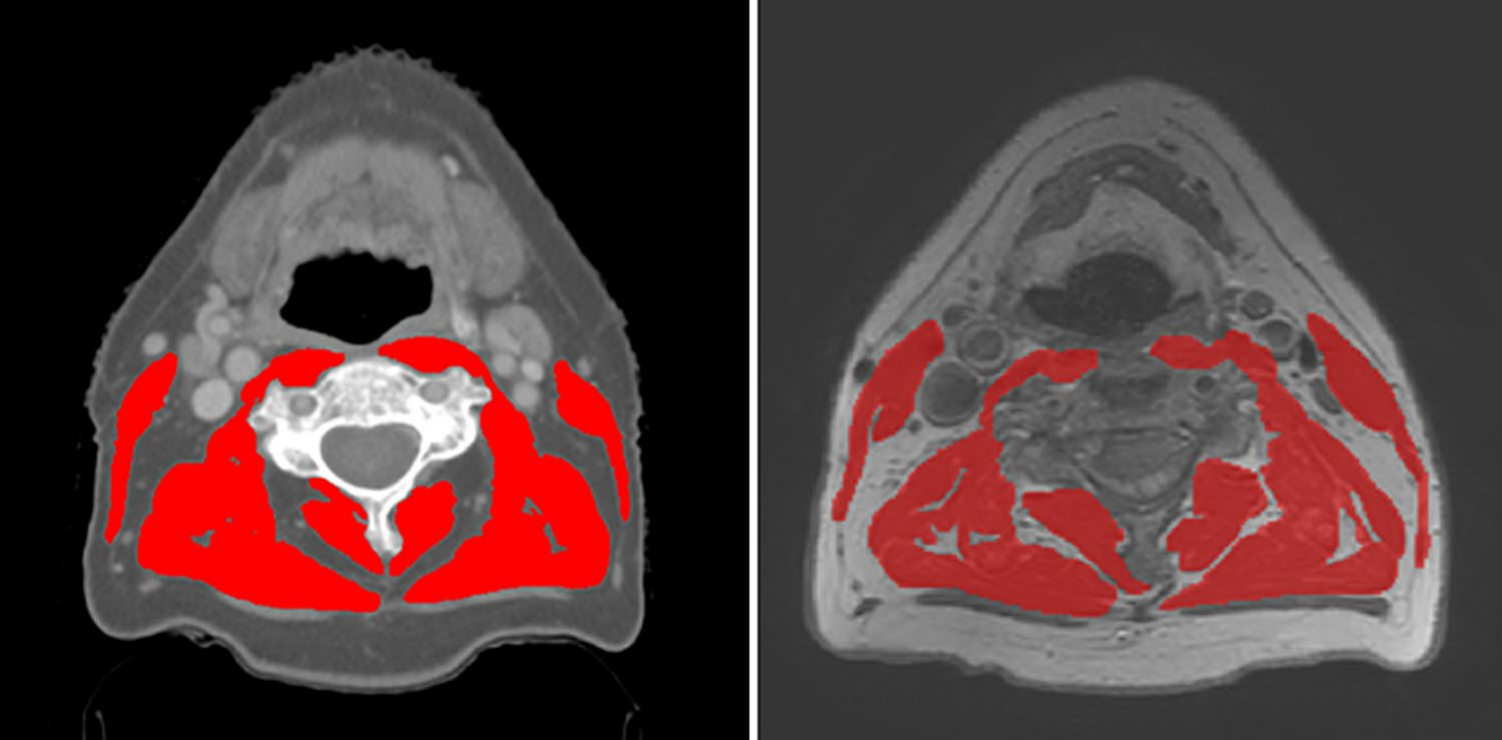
Example of delineation on 3 mm axial slide of CT (Siemens 40-slice) (left) and axial T1 weighted sequence MRI (Philips 1.5 T) (right) at the level of C3 using SliceOmatic. The left and right SCM as well as the paravertebral muscles are delineated excluding the trapezius muscle. Please note that the muscles in the anterior neck are not included in the delineation as previously described
